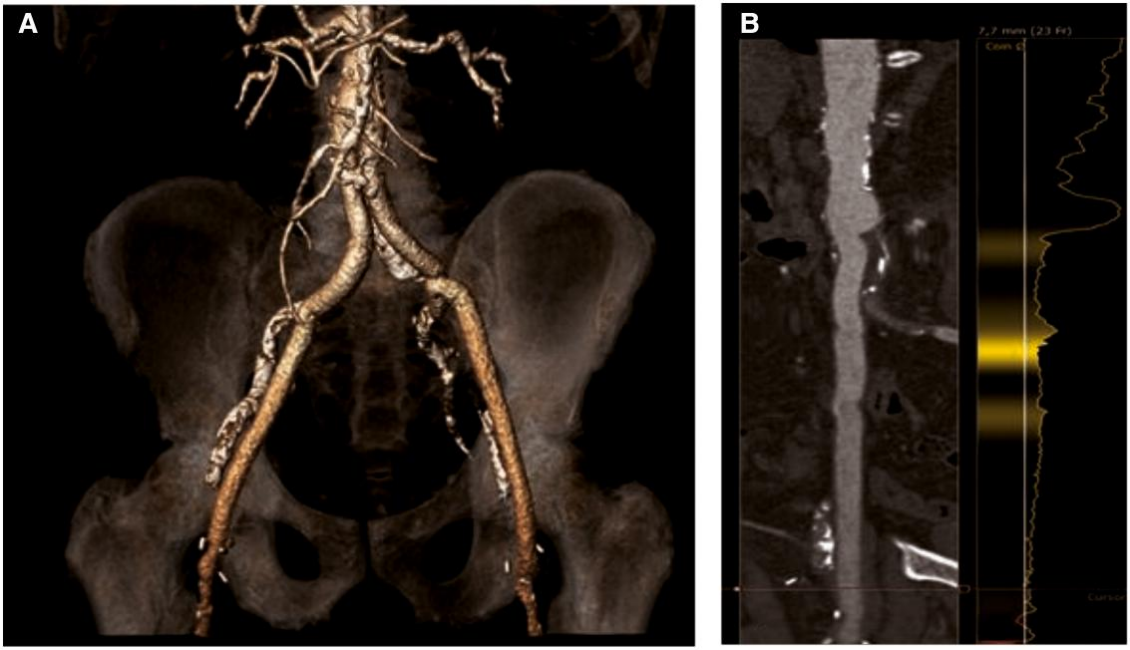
(A)* Multislice CT (three-dimensional reconstruction) shows the bilateral aortoiliac graft and the femoral arteries. *(B)* Sufficient luminal diameter of the (right) Y-graft.
